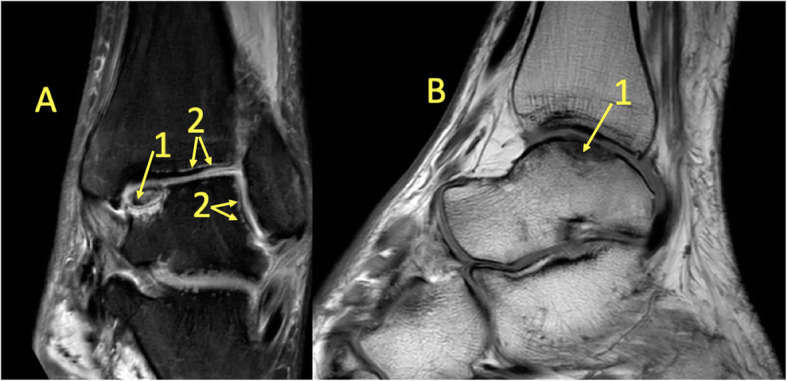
A 29-year-old male with 4-month history of medial ankle pain and suspicion of an osteochondral lesion. (1) MRI showed a stable non-displaced osteochondral lesion and (2) subchondral BME, consistent with early inactive osteopenia
